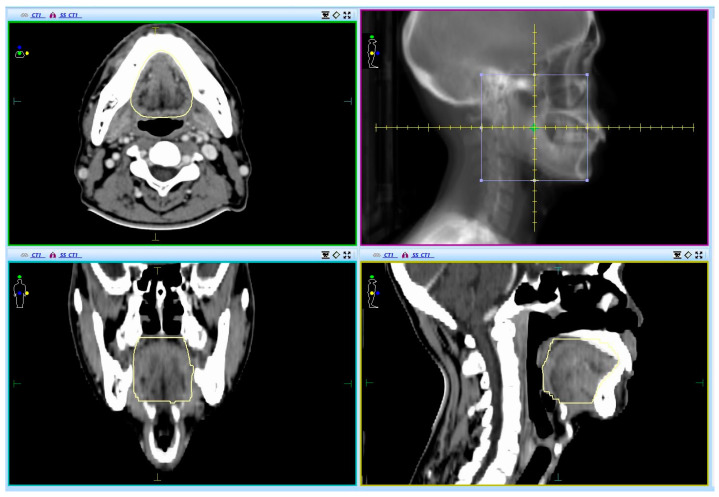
Delineation of the oral cavity in computed tomography scan.
